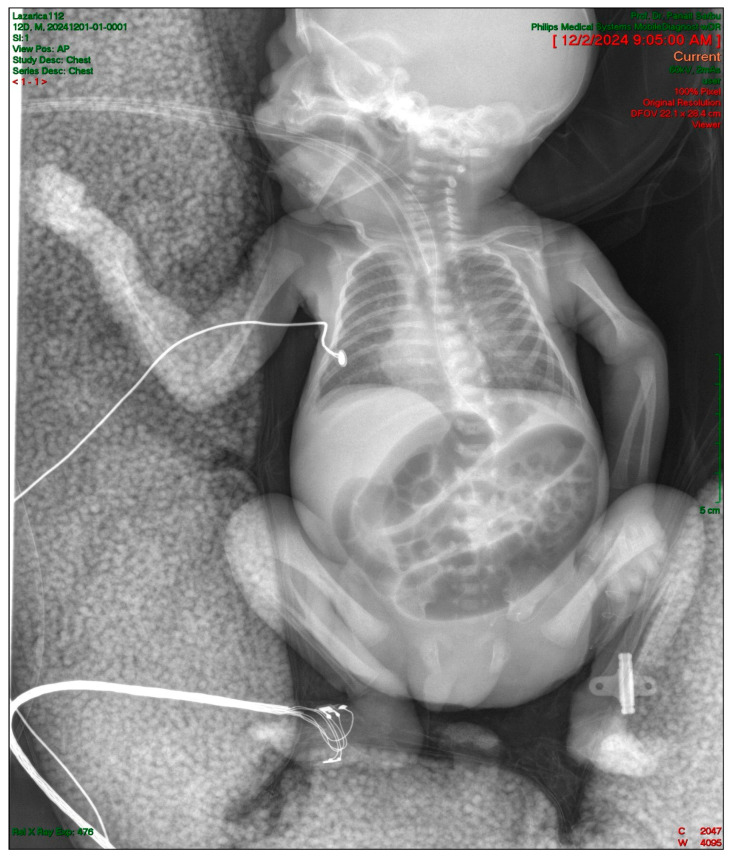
Abdominal X-ray demonstrating significantly dilated intestinal loops, with absence of gas in pelvic region, suggesting distal intestinal obstruction. This radiographic finding, combined with clinical symptoms of feeding intolerance and absent stool passage, prompted urgent surgical consultation for suspected intestinal obstruction.
